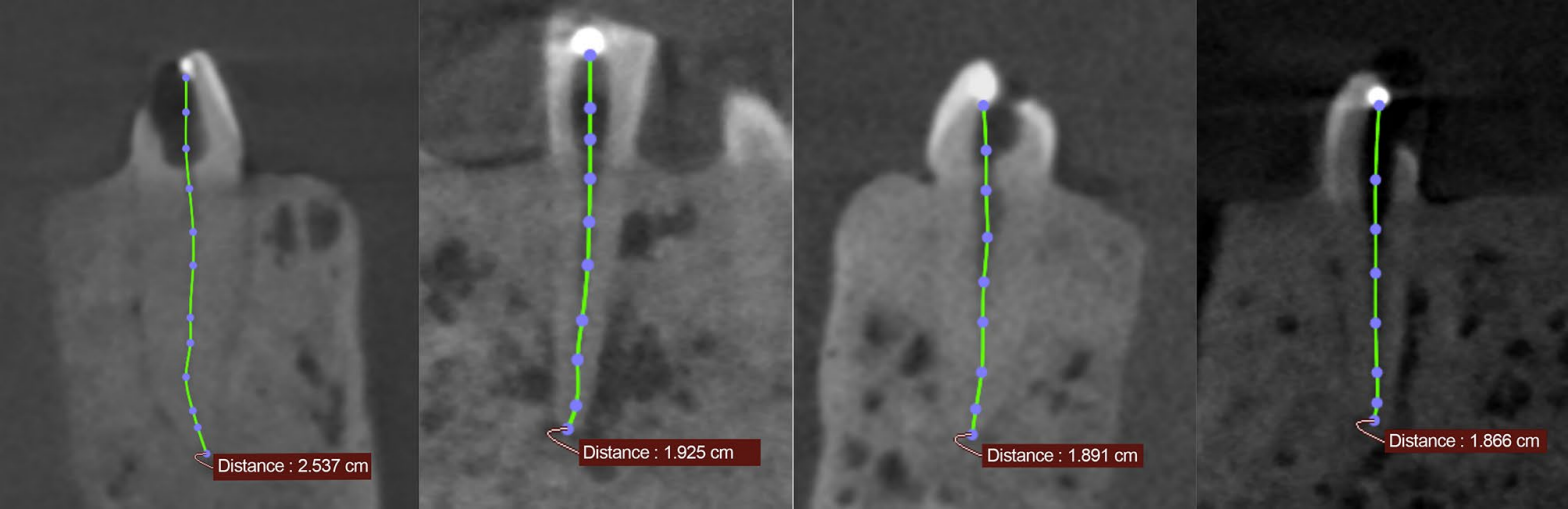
Resulting (exemplary) CBCT scans with the polygon measurement tool.
